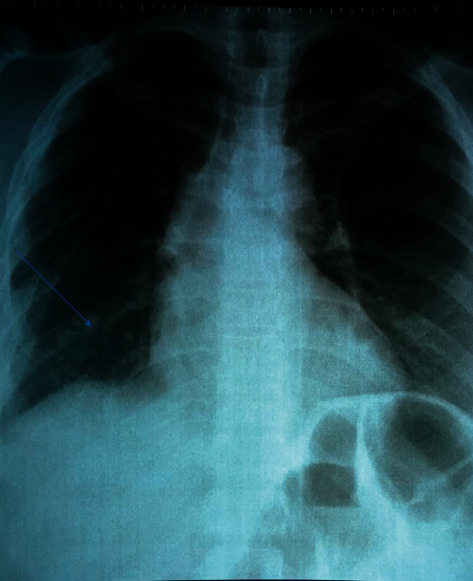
A plain chest radiograph of the study subject in anterioposterior view showing a near total re-expansion of the lung after chest tube drainage.
